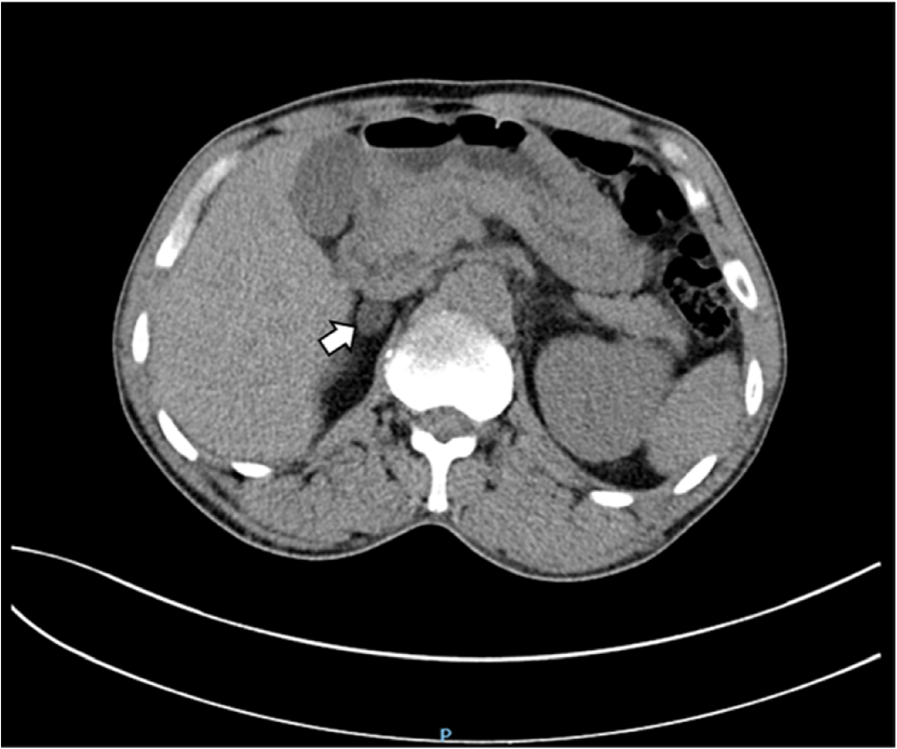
Plain computed tomography scan shows mass in the right adrenal gland. Arrow: right adrenal tumor
